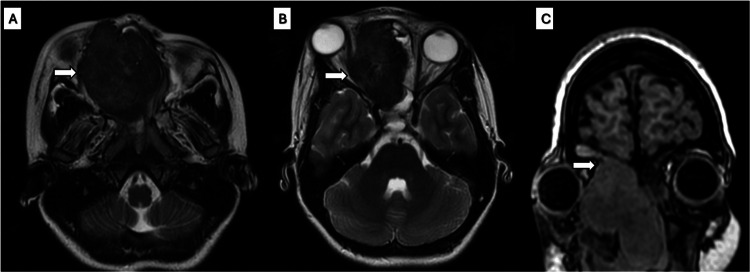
Case 2: Preoperative MRI A) Axial T1-weighted image demonstrating a heterogeneous expansile mass in the right nasal cavity displacing the right orbit laterally (white arrow). B) Axial T2-weighted image showing orbital involvement (white arrow). C) Coronal T1-weighted image demonstrating the involvement of the anterior cranial fossa (white arrow).
